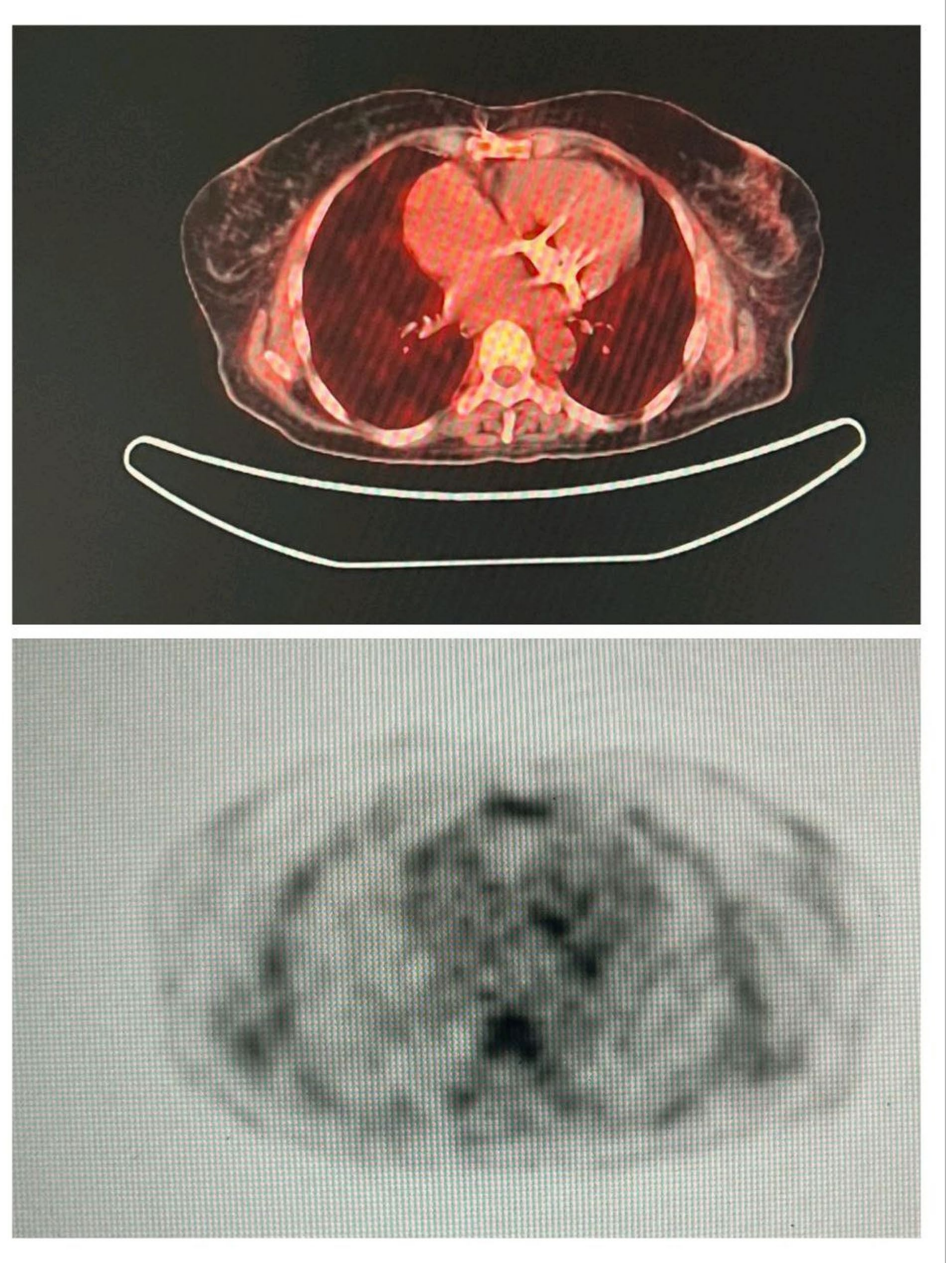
FDG-PET scan demonstrating pathological uptake in bone, consistent with metastatic involvement
